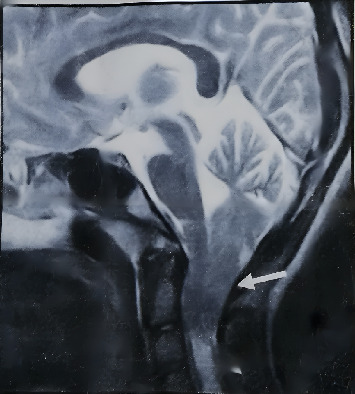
MRI of the brain. MRI of the brain showing downward displacement of the cerebellar tonsils below the foramen magnum, resulting in narrowing and compression of the craniocervical junction and upper spinal cord.
